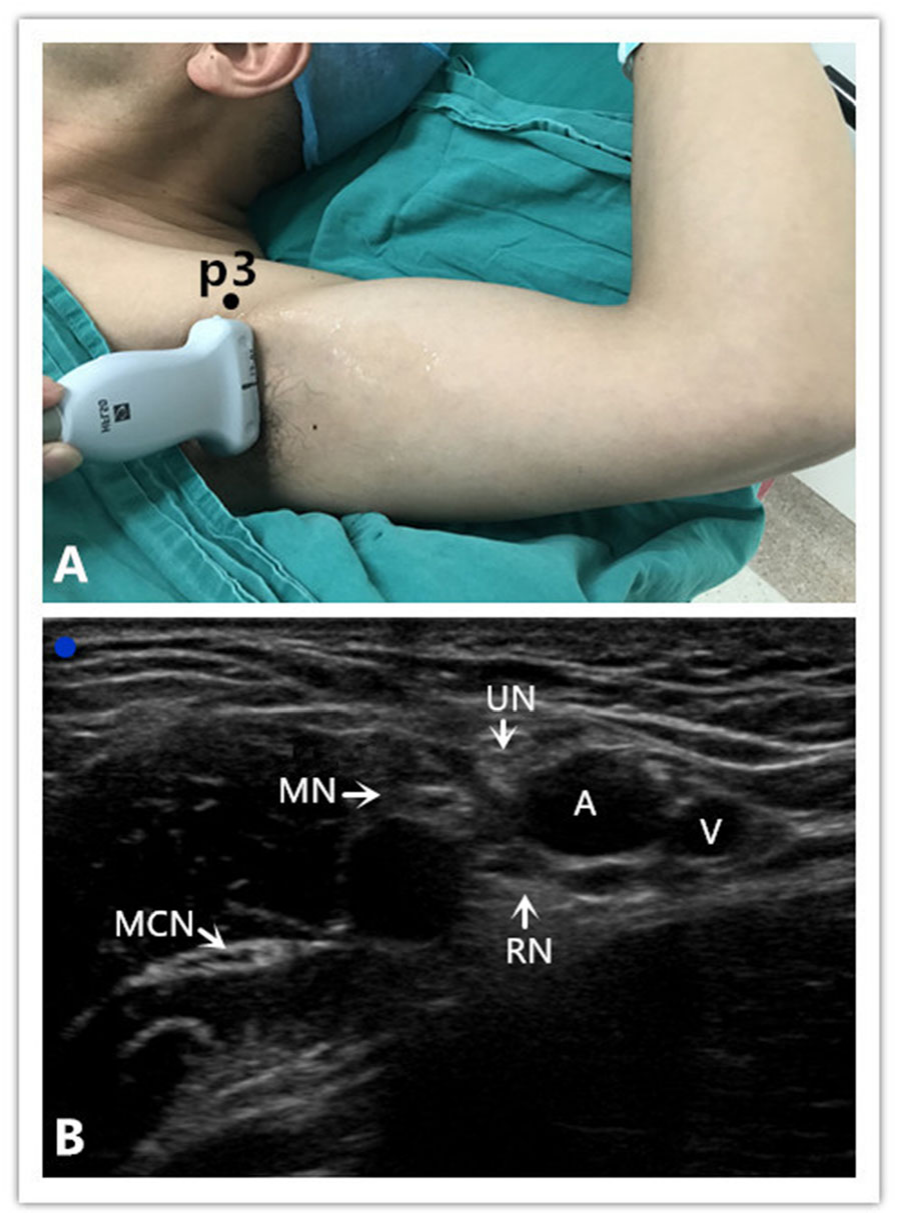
Position of patient and ultrasound imaging in the operating room when performing the axillary block. a Position of the patient, the probe, and the needle (“p3”) during the axillary block. b Ultrasound depiction of the axillary. Radial nerve (RN), musculocutaneous nerve (MCN), median nerve (MN), ulnar nerve (UN), axillary artery (A) and axillary vein (V) The blue point is situated at the lateral side of the probe
